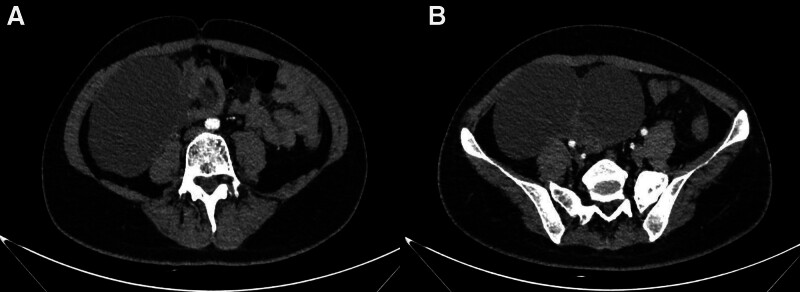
November 28, 2021. Pelvis computed tomography: bilateral cystic solid masses in the adnexal region, considered to be of high malignant potential, with possible cystic adenocarcinoma. Pelvic and abdominal fluid accumulation. (A) Pelvic metastasis. (B) Maximum cross-section of pelvic metastases.
